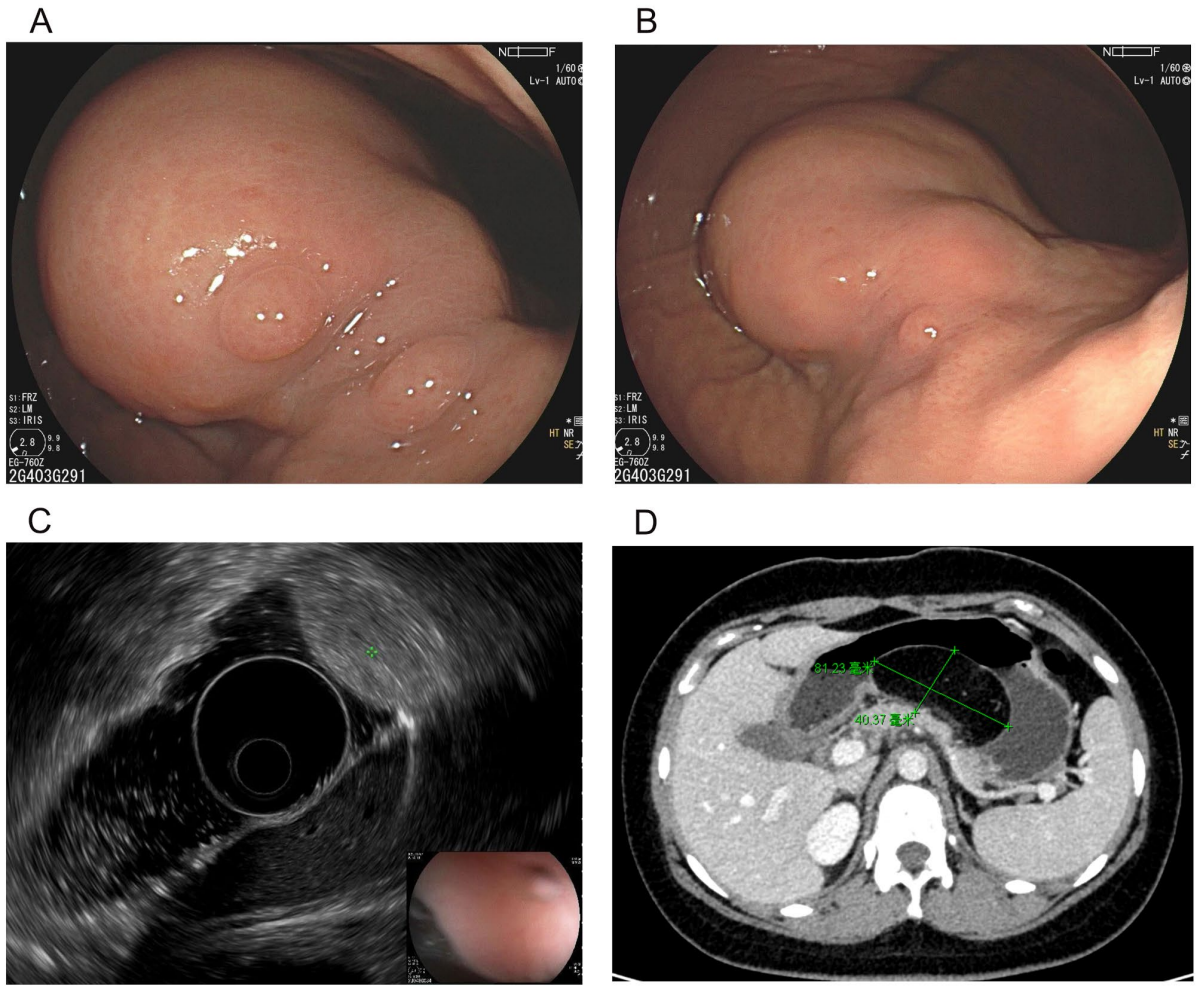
A large, smooth, soft mass in the stomach’s body can obstruct the lumen when the stomach is not fully expanded (A). When inflated, the mass appears to have a broad base (B). EUS demonstrates uniform high echogenicity (C), and CT reveals an 8.12 cm fatty density within the wall of the gastric body (D)
